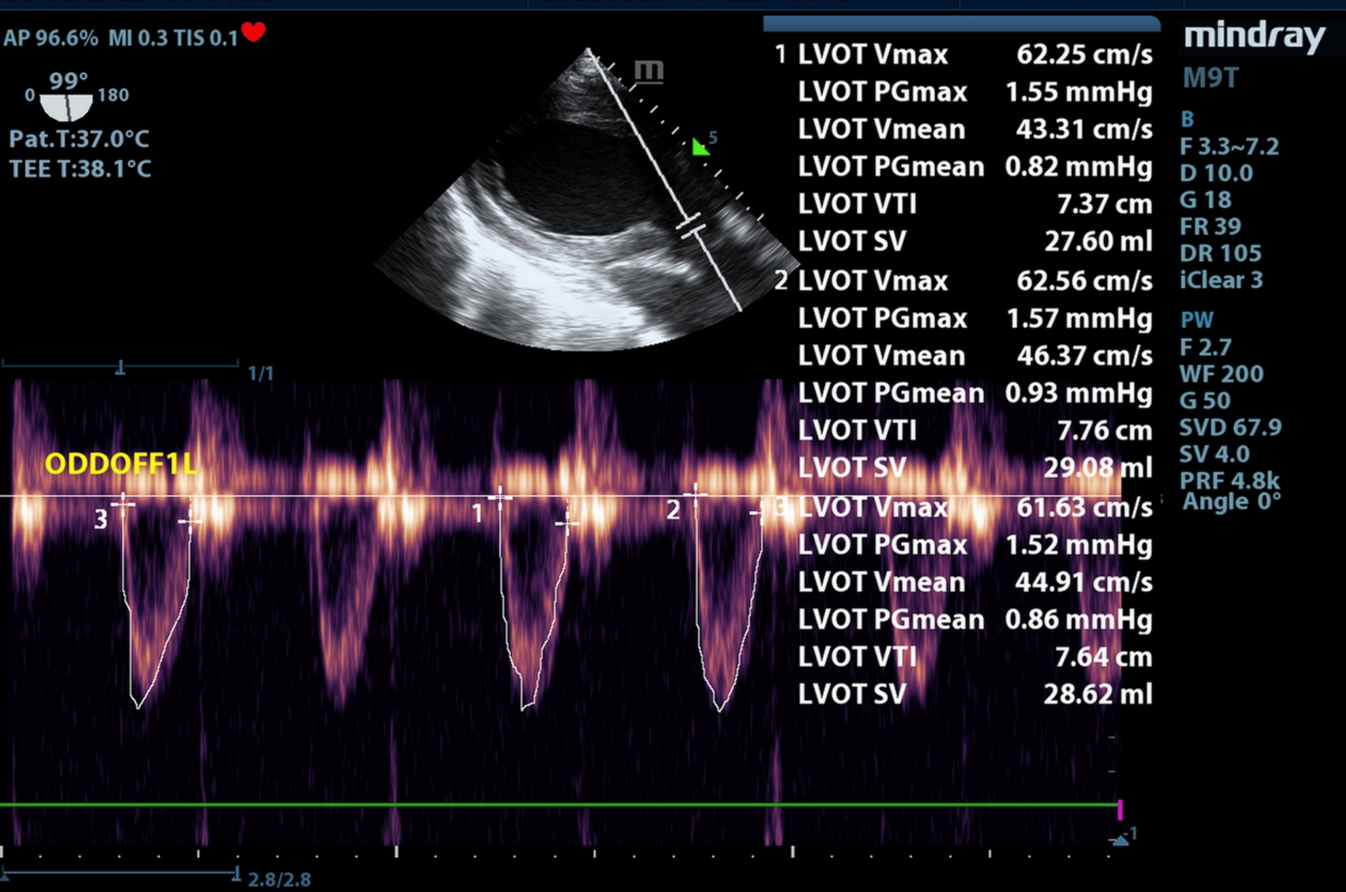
Transgastric long-axis view Pulse wave (PW) Doppler at the left ventricular outflow tract (LVOT), used to calculate LVOT velocity time integral (VTI) and stroke volume (SV). The heart rate is 75 bpm, with a calculated SV of 28.7 mL and a cardiac output (CO) of 2.1 L/min. These findings are consistent with low CO and cardiogenic shock.
